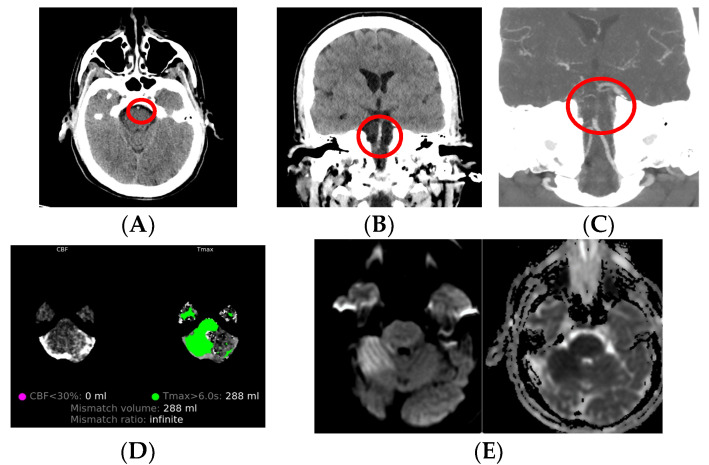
Male patient, 45 years old, presented with vertigo and disorientation at the emergency department, after which a neurologist ordered neuroimaging. (A,B) Non-contrast CT shows hyperdense basilar artery (red circle). (C) CT angiography shows occlusion of basilar artery. (D). CT perfusion shows mismatch volume of 288 mL and marks ischemia zone. (E) MRI in sequences DWI and ADC show restricted diffusion in the right cerebellar region, marking acute ischemia.
